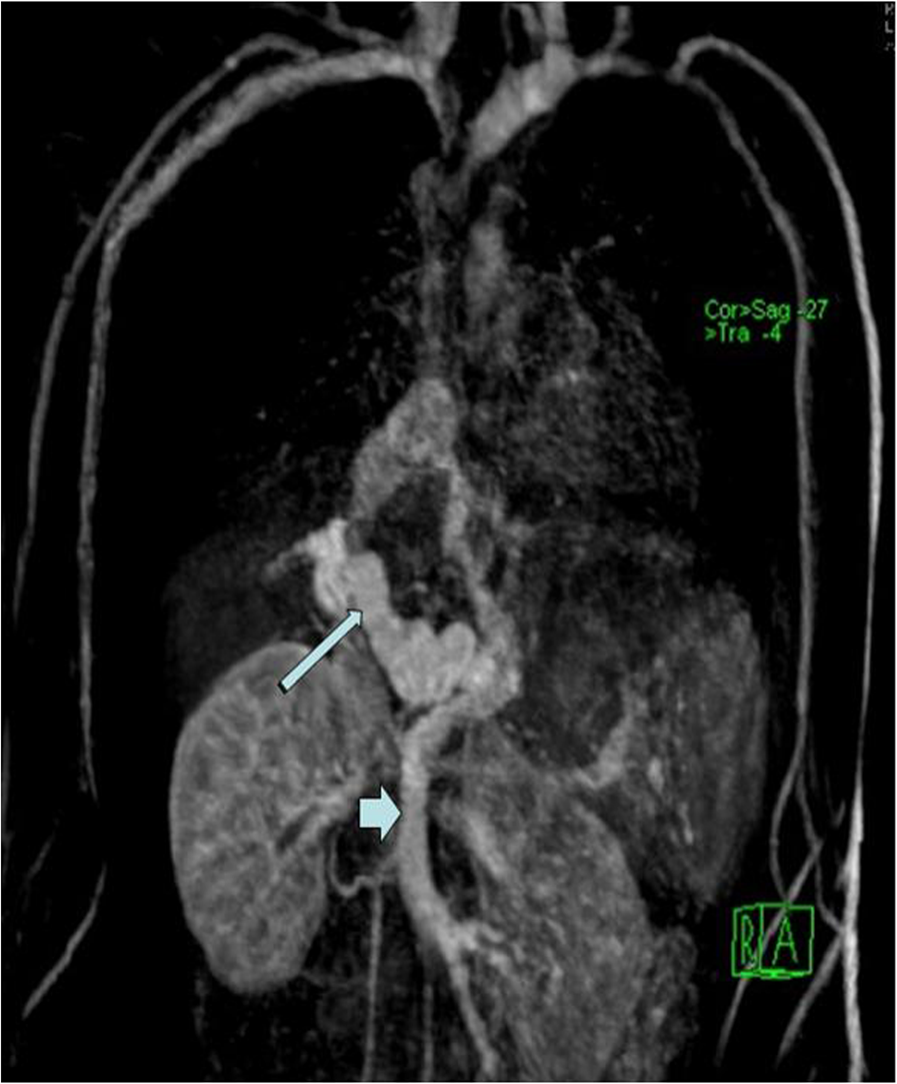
Coronal magnetic resonance imaging (MRI) scan of the chest and abdomen section: dilated portal vein (long arrow) and congested mesenteric veins (short arrow).
